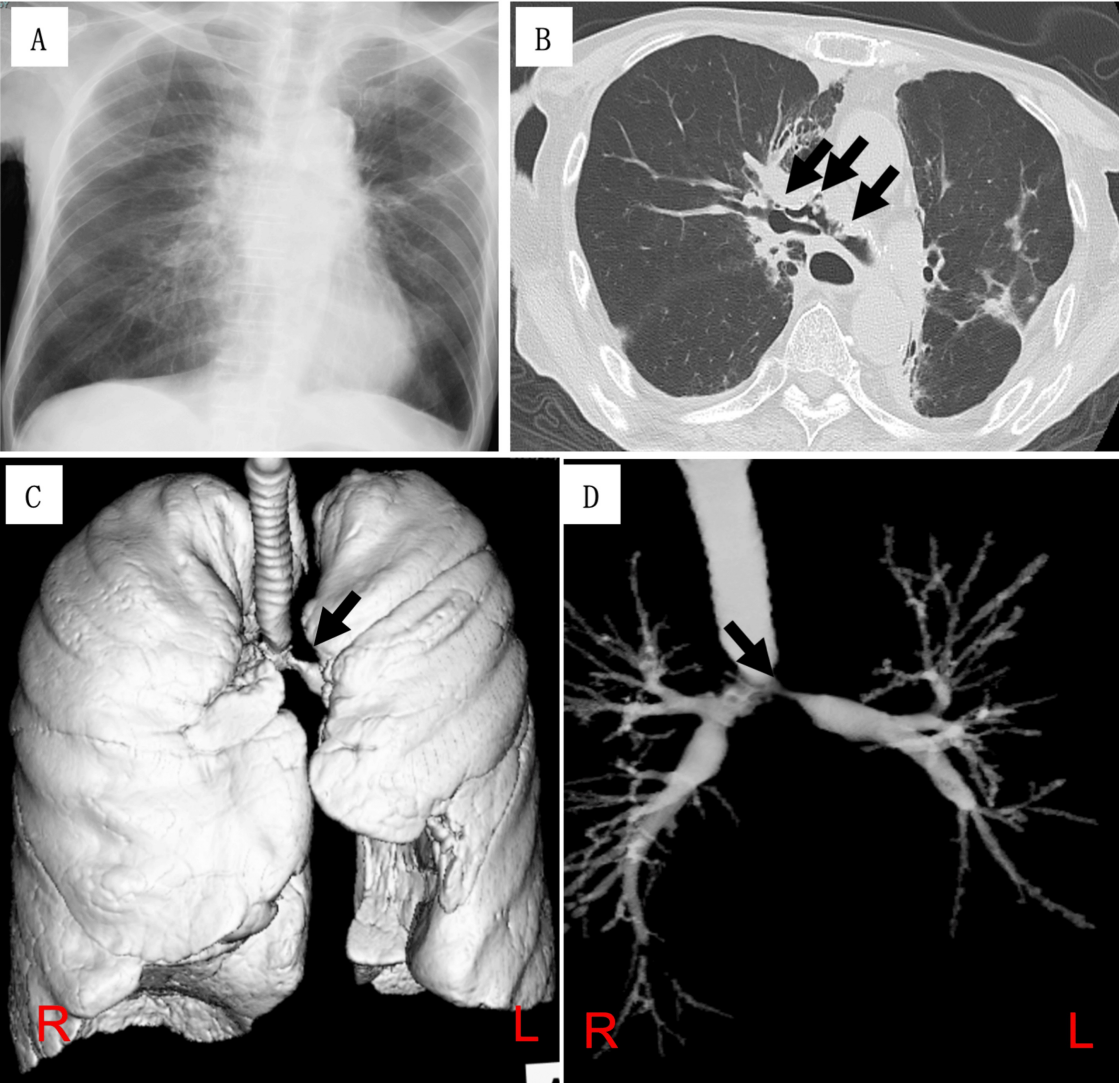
Imaging findings at admission (A) Chest X-ray showing a linear shadow and ground-glass opacity in the left upper lung field, with no significant changes compared to three months prior; (B) Chest CT revealing marked stenosis of the tracheal bifurcation and bilateral main bronchi, with extratracheal air (arrows); (C) 3D imaging (VR) demonstrating marked stenosis of the left main bronchus (arrow); (D) Isolated tracheal imaging showing more pronounced stenosis of the left main bronchus (arrow). R and L indicate the right and left sides, respectively.
